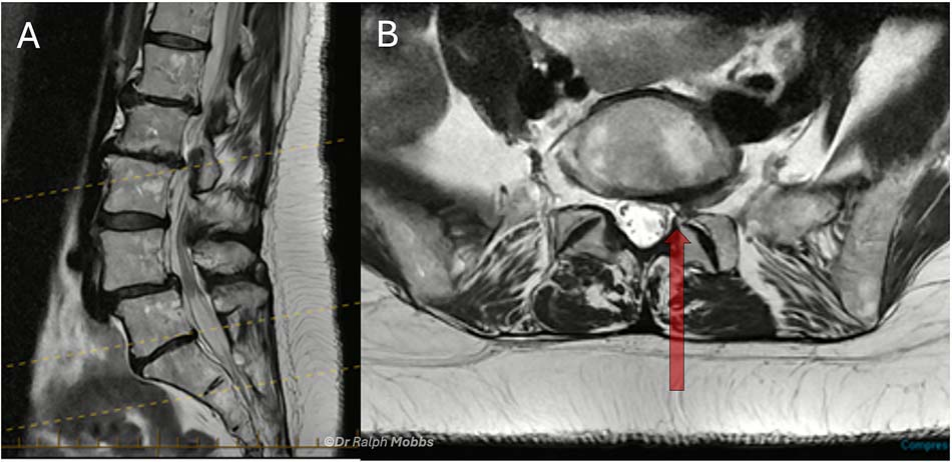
Case 2 mid-sagittal (A) and axial (B) *T*_2_-weighted MRI at L5/S1 with lateral recess stenosis (arrow).
